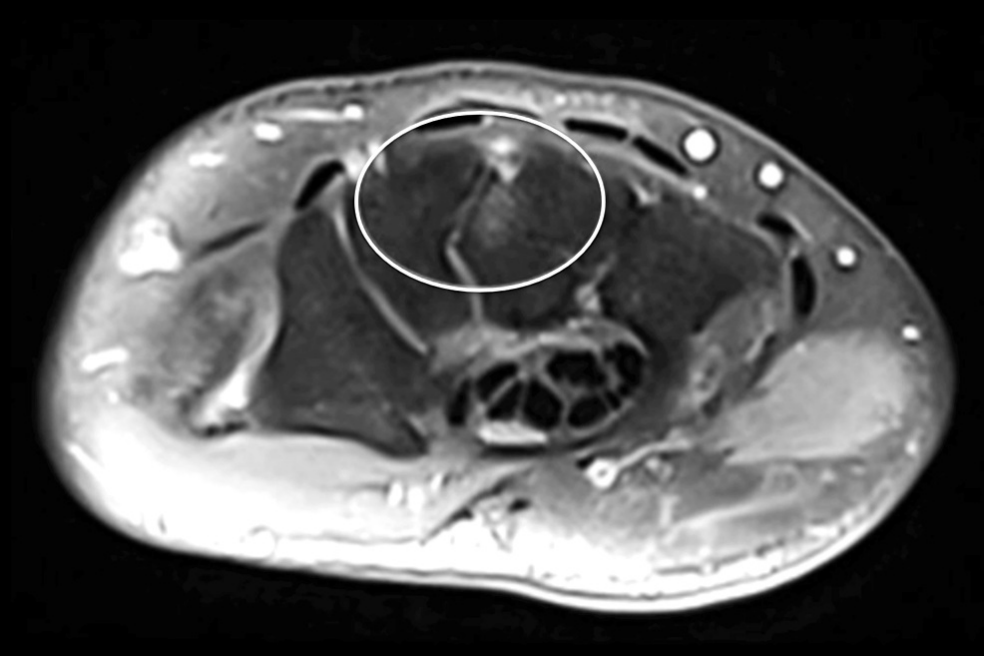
PD-weighted axial MRI image of the left wrist showing the abnormal fibrous coalition (encircled) between the capitulum and the trapezoid. PD: proton density; MRI: magnetic resonance imaging.
